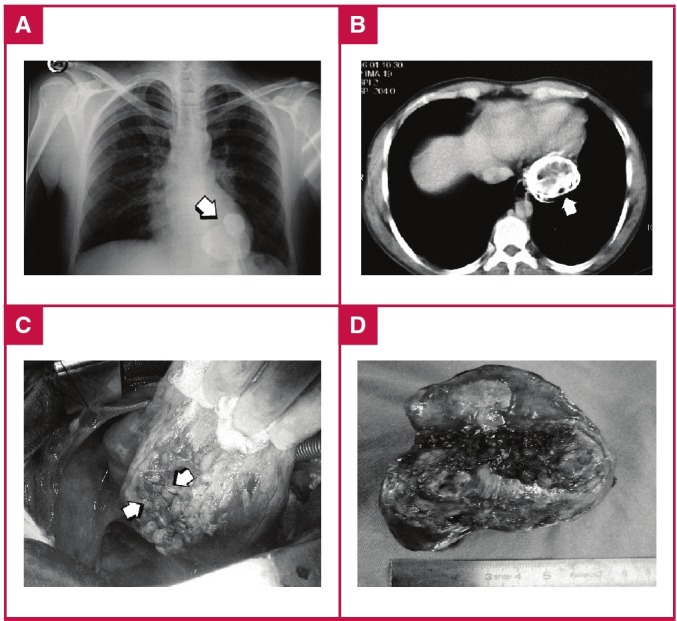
A. Plain chest X-ray showing the calcified outer layer of the cardiac hydatid cyst. B. Eggshell appearance of the cardiac hydatid cyst located on the posterior left ventricular wall on computerised tomography. C, D. Surgically closed defect after removal of the highly calcified hydatid cyst.
